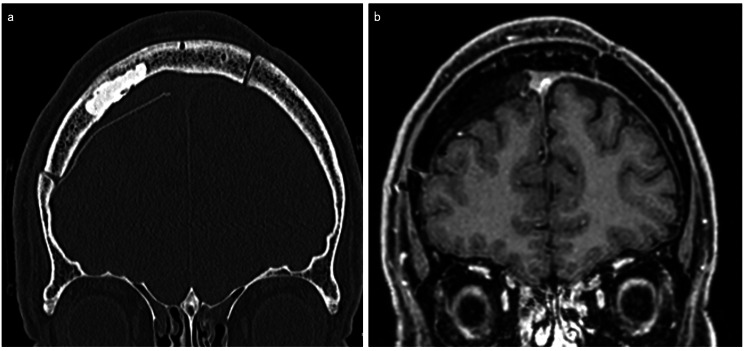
Postoperative CT scan and contrast MRI (a) CT scan taken nine months after surgery showed no sign of bone paste falling off; (b) Contrast MRI performed one year after surgery showed no recurrence in the cranioplasty area
